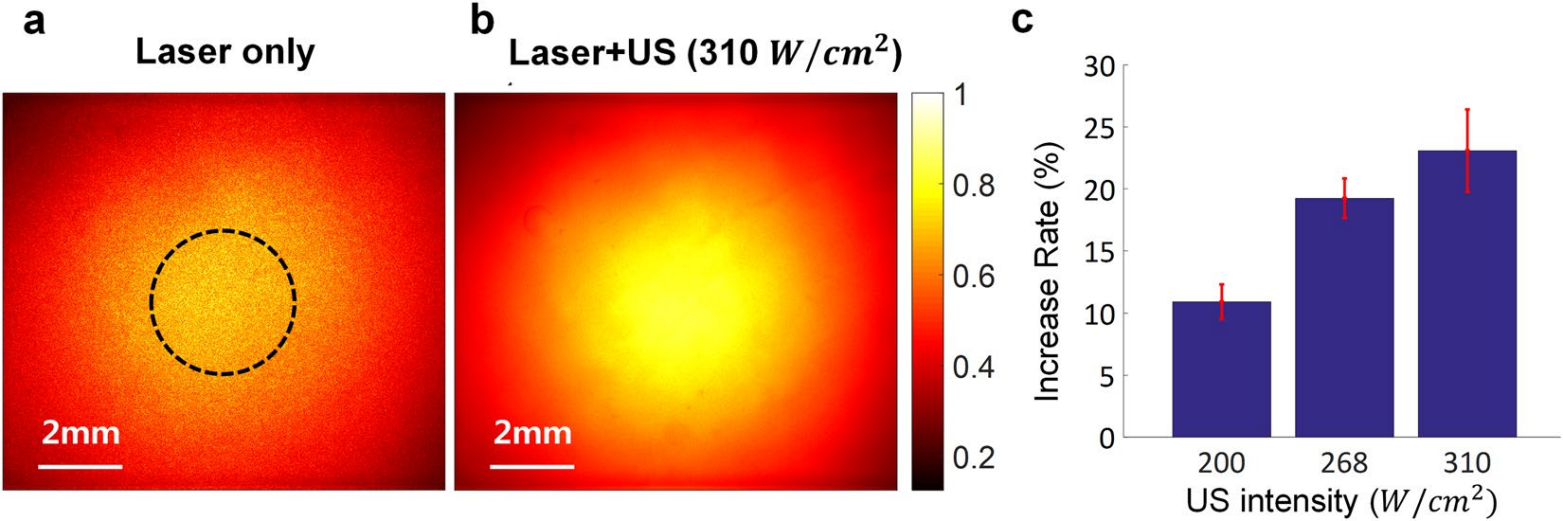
Measurement of light intensity distribution on the *ex vivo* chicken breast with a thickness of 7 mm. (a) Light intensity distribution measured at the end of the chicken breast by a CCD camera when laser irradiation only was delivered and (b) both laser and ultrasound (US) with an intensity of 310 W/cm^2^ were delivered into the medium. (c) Average light intensities at each ultrasound intensity and the rate of intensity increase from the intensity measured when only the laser was transmitted. The ROI within which the average normalized intensity was measured is indicated by a black dashed circle.
